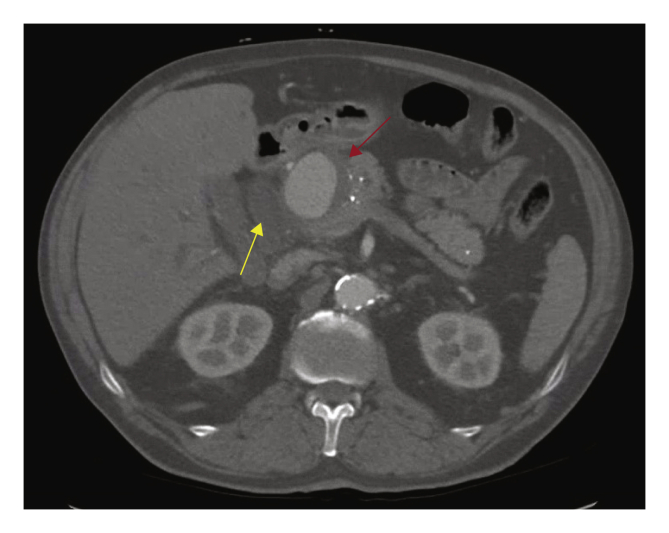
Computed tomography scan before intervention. Yellow arrow: dilated common bile duct, red arrow: true aneurysm of the common hepatic artery.
